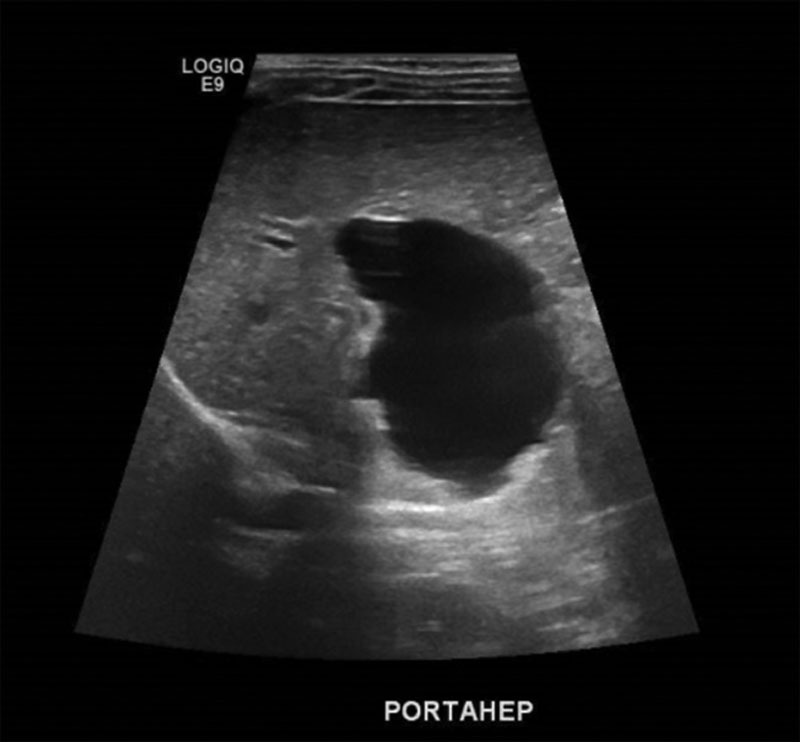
Ultrasonography of the abdomen showing well defined cystic area at the porta hepatis.
